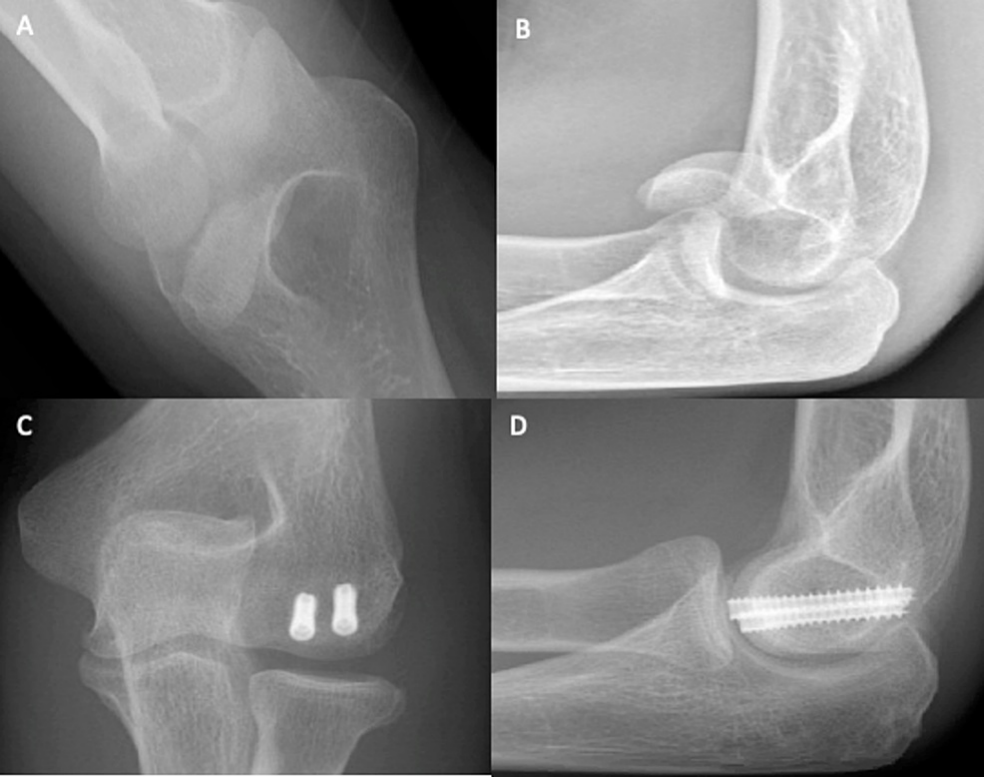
Plain elbow X-rays of case 2. Figure 2A, 2B shows anteroposterior and lateral views, respectively, of a type IV capitellum fracture. Figure 2C, 2D shows anteroposterior and lateral views, respectively, of the elbow after ORIF. ORIF: open reduction and internal fixation
